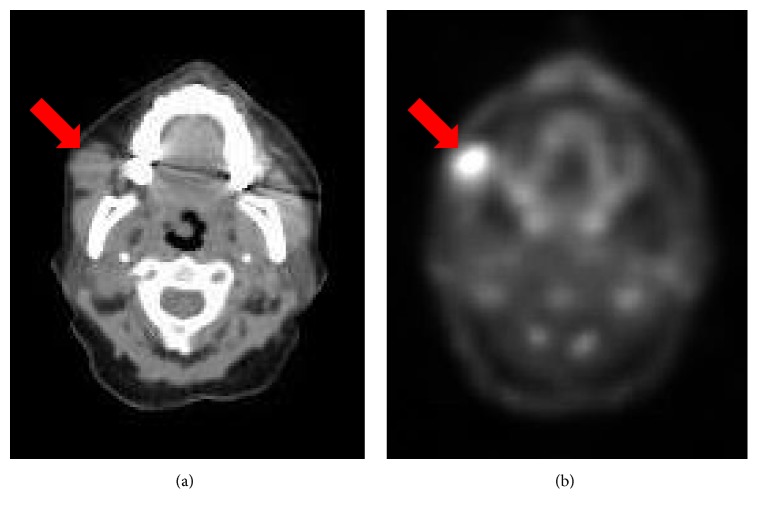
CT (a) and PET (b) images of the FDG-avid mass, centered along the anterior aspect of the right masseter muscle, not involving parotid.
